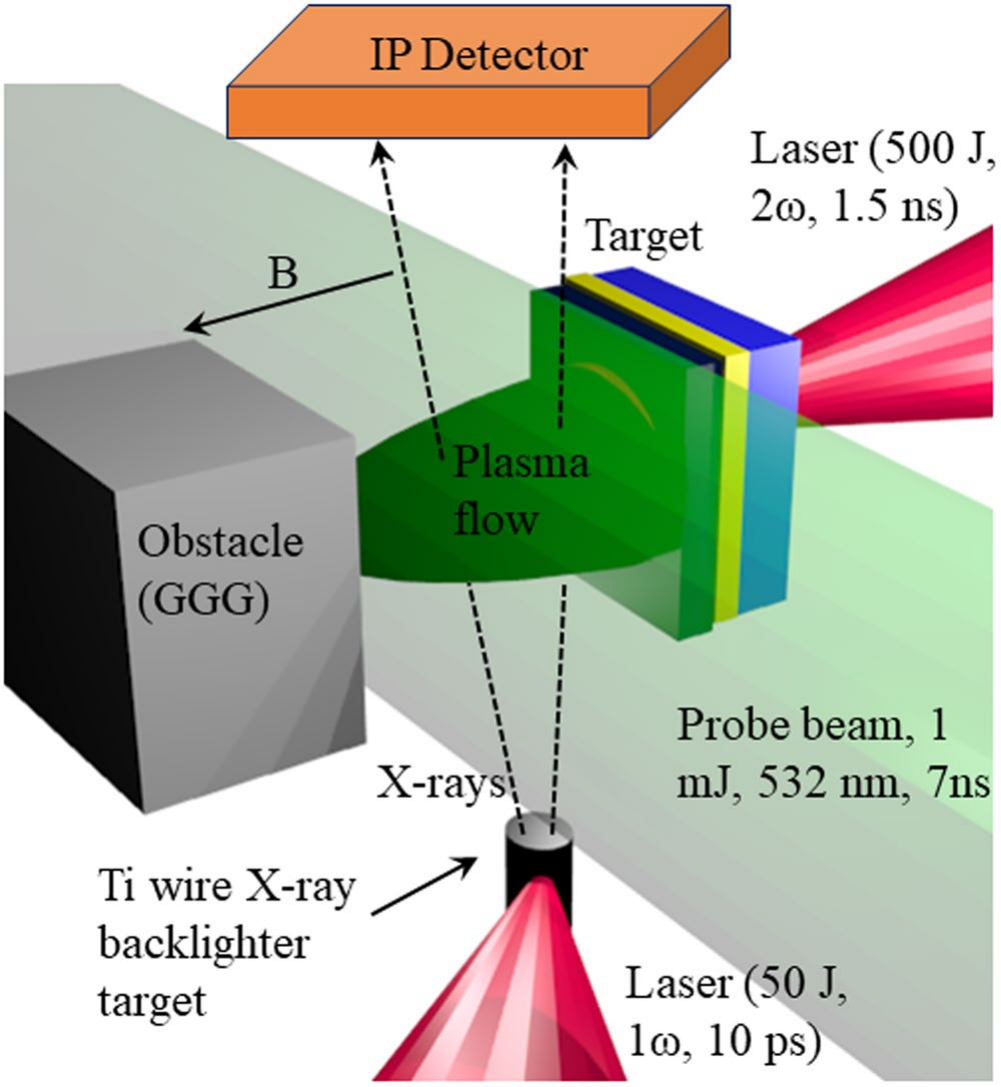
Experimental setup at the LULI2000 laser facility. The drive laser, interacts with the multi-layer target, creating a plasma flow travelling towards the obstacle. The interaction of a short-pulse laser with a wire target generates X-rays which are used to radiograph the plasma flow, with an image plate detector. An optical probe beam, orthogonal to the plasma flow is used to perform Schlieren imaging, and self-emission of the experiment is recorded on the same axis. A constant, homogeneous magnetic field of 15 T in the direction of the plasma flow is applied across the whole system.
